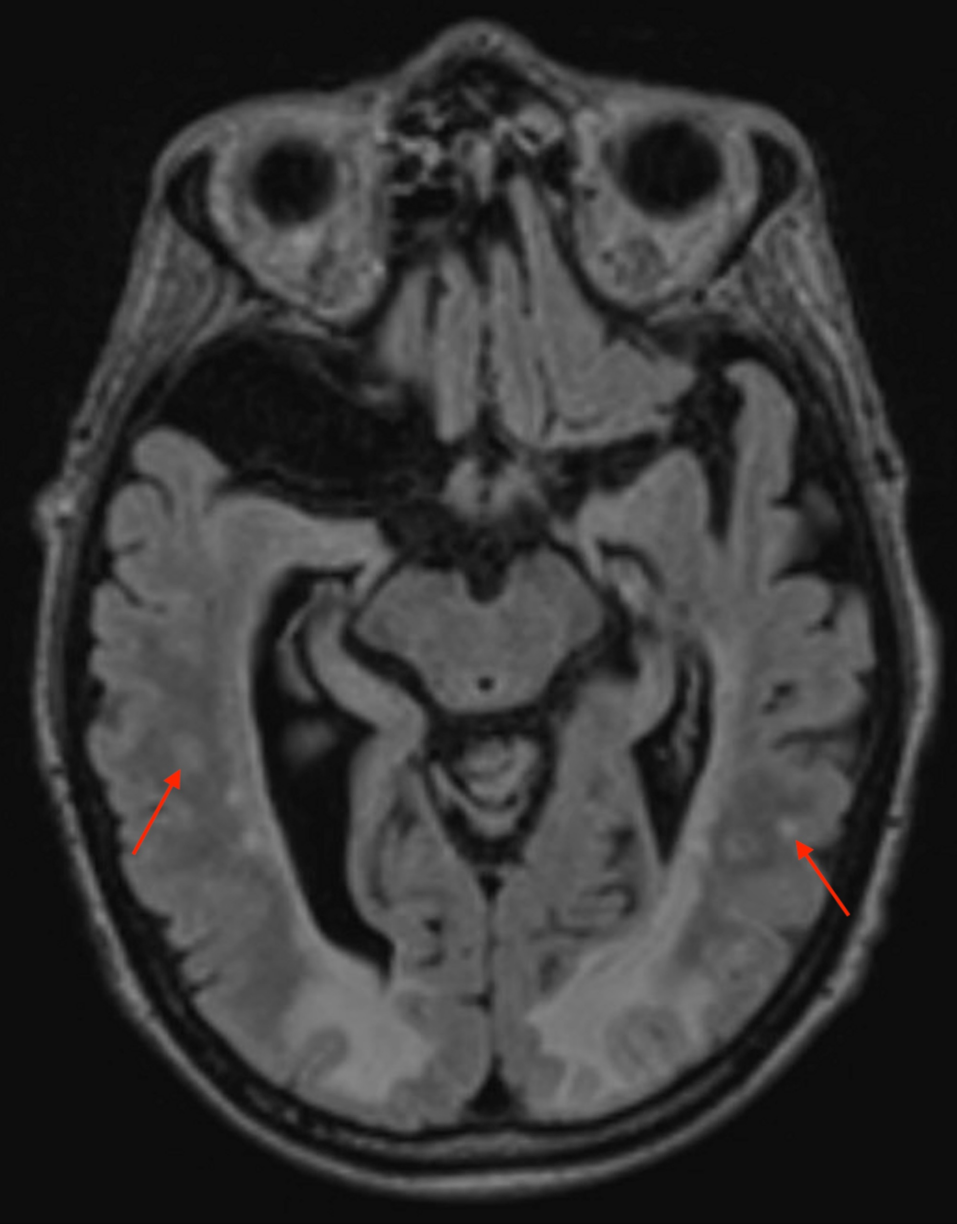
Fluid-attenuated inversion recovery (FLAIR) sequence MRI brain representing established small vessel ischaemic changes.
